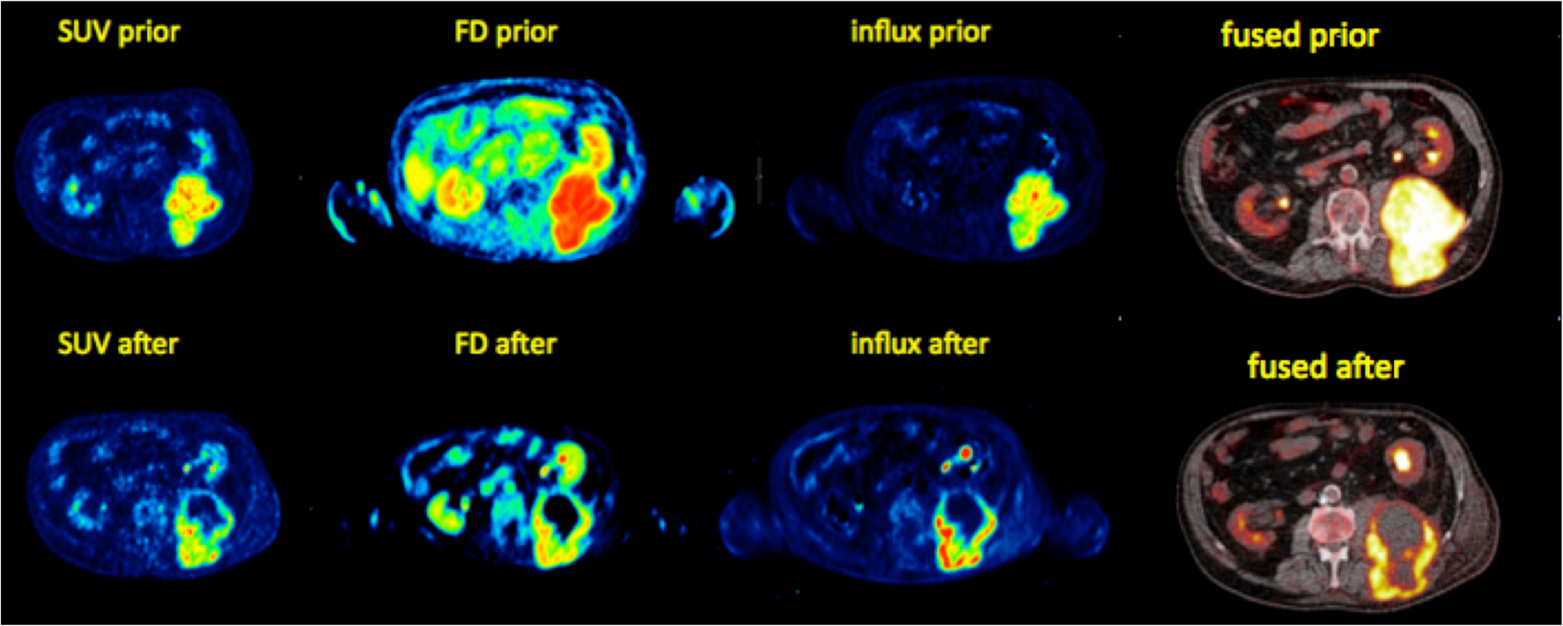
Patient with an advanced high-grade retroperitoneal sarcoma infiltrating the left dorsal muscles. Tranversal ^18^F-FDG PET images prior (upper row) and after the end of neoadjuvant pazopanib therapy (lower row) on the left part of the figure. The 55–60-min SUV images demonstrate a decrease of uptake and a central tumor necrosis as response to therapy (left). Comparable ^18^F-FDG response pattern in the parametric images of the fractal dimension and the influx according to Patlak (middle). “Conventional,” tranversal-fused PET/CT images on the right part
